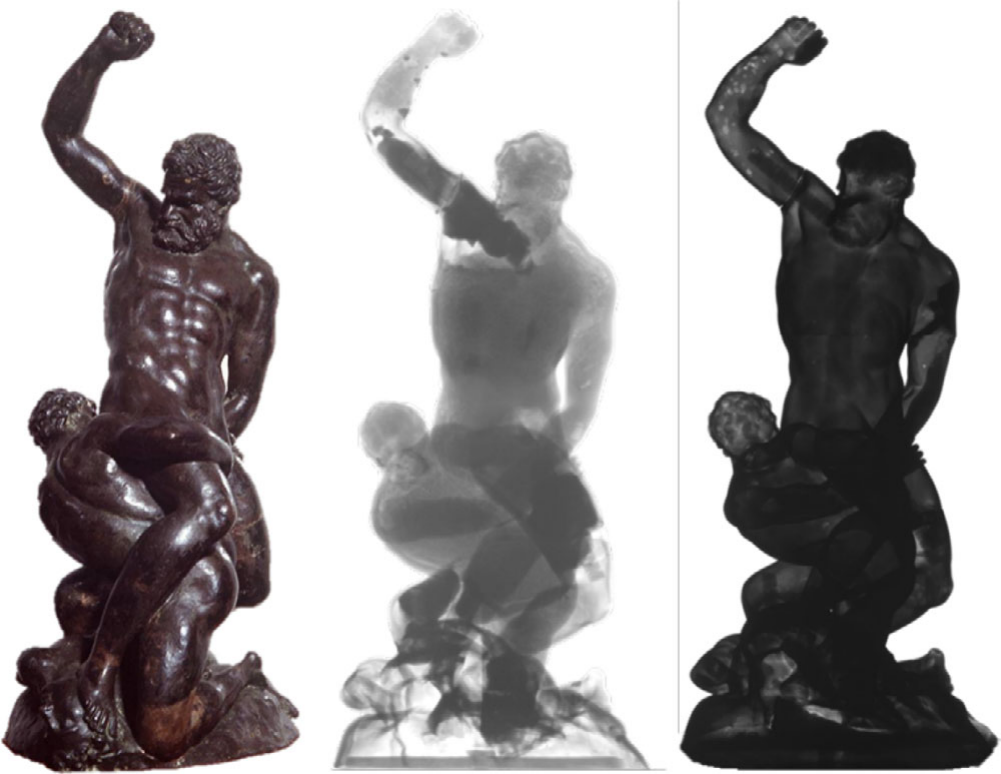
A multimodal view of the sculpture *Samson Slaying a Philistine*. Pictured are (left) a visual reference photograph, (centre) a neutron radiograph, and (right) an X‐ray radiograph. The neutron image reveals organic fillers and restoration materials, while the X‐ray image highlights dense metal, showing casting defects and internal supports. Together, they provide a comprehensive, non‐invasive understanding of the object's structure and history. Reproduced from Ref. 62 under CC BY 3.0
